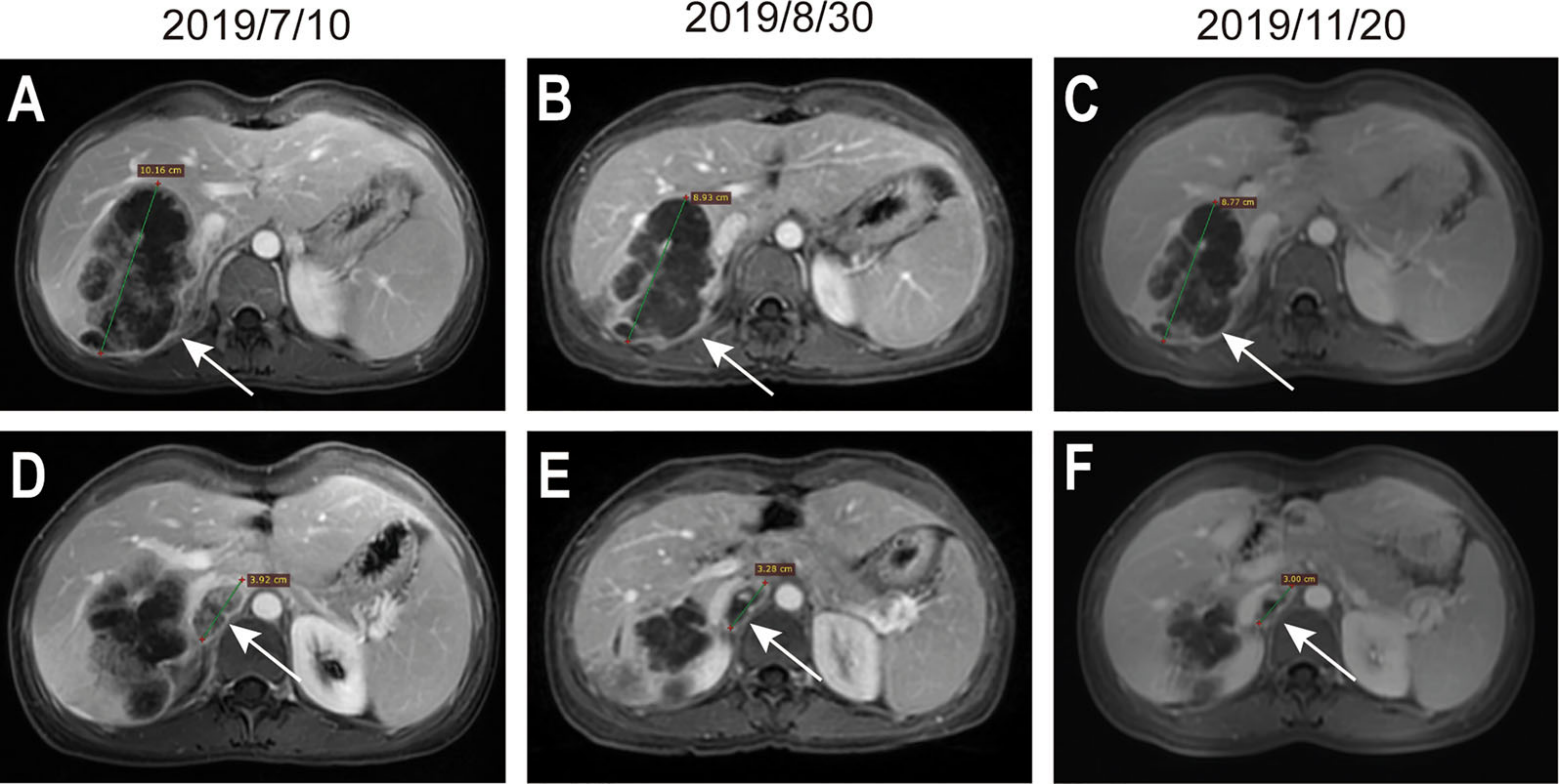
MRI evaluation during preoperative systemic treatment. (A–C) indicate the maximum diameter of the lesion located in the liver, the maximum diameter of the lesion in (A–C) were 10.16cm, 8.93cm, and 8.77cm. (D–F) indicate the lesion located in the tumorigenic thrombus of the inferior vena cava, the maximum diameter of the lesion in (D–F) were 3.92cm, 3.28cm, and 3.00cm. Arrows in the figures indicate the position of lesions.
